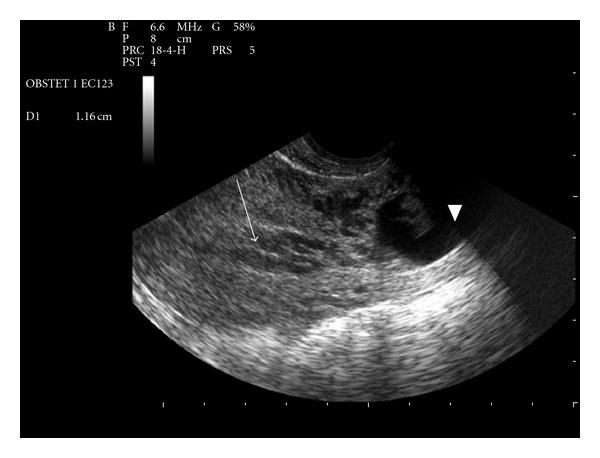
Transvaginal ultrasound image of the scar pregnancy. Gestational sac in the lower anterior wall of the uterus (arrow head), empty uterus (arrow).
